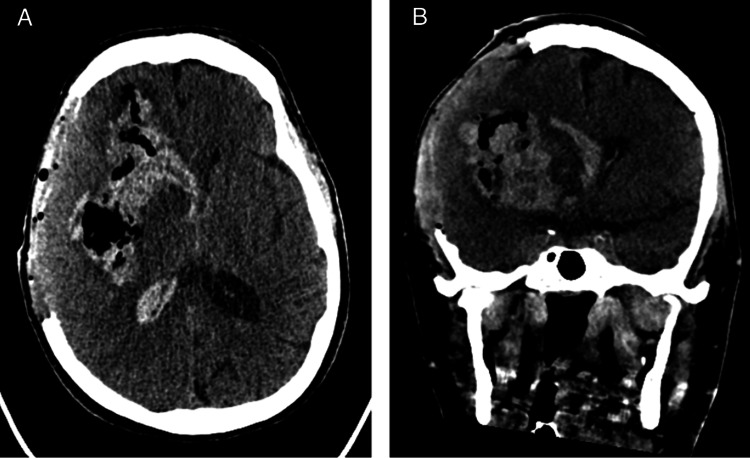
Axial (A) and coronal (B) post-operative CT of the head following right-sided decompressive hemicraniectomy.
